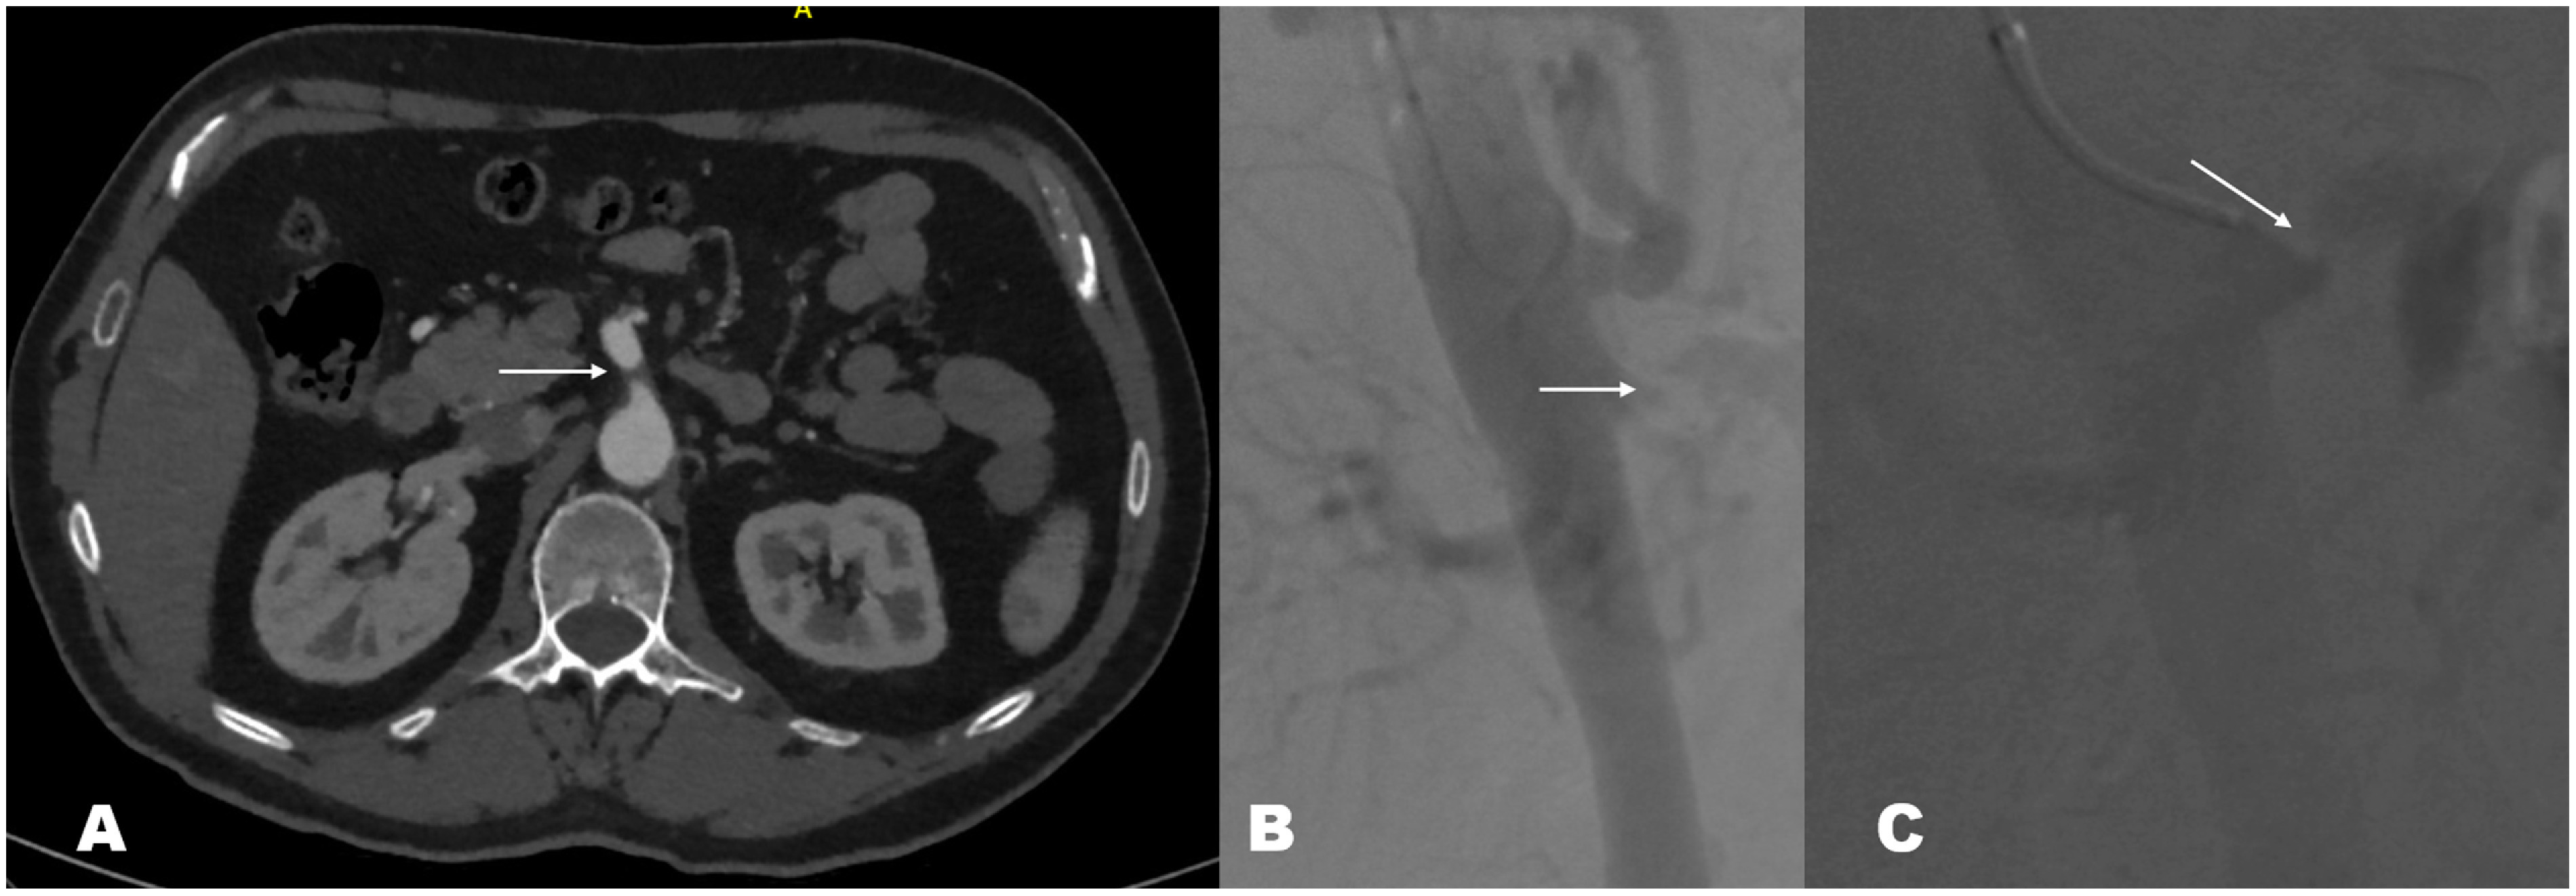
Fig. 2 (A) Computed tomography angiography showing a stenosed superior mesenteric artery with distal perfusion. There is no embolus nor atherosclerotic changes surrounding the lesion. (B) Nonselective angiography showing isolated superior mesenteric artery dissection (ISMAD). (C) Selective angiography showing ISMAD.
